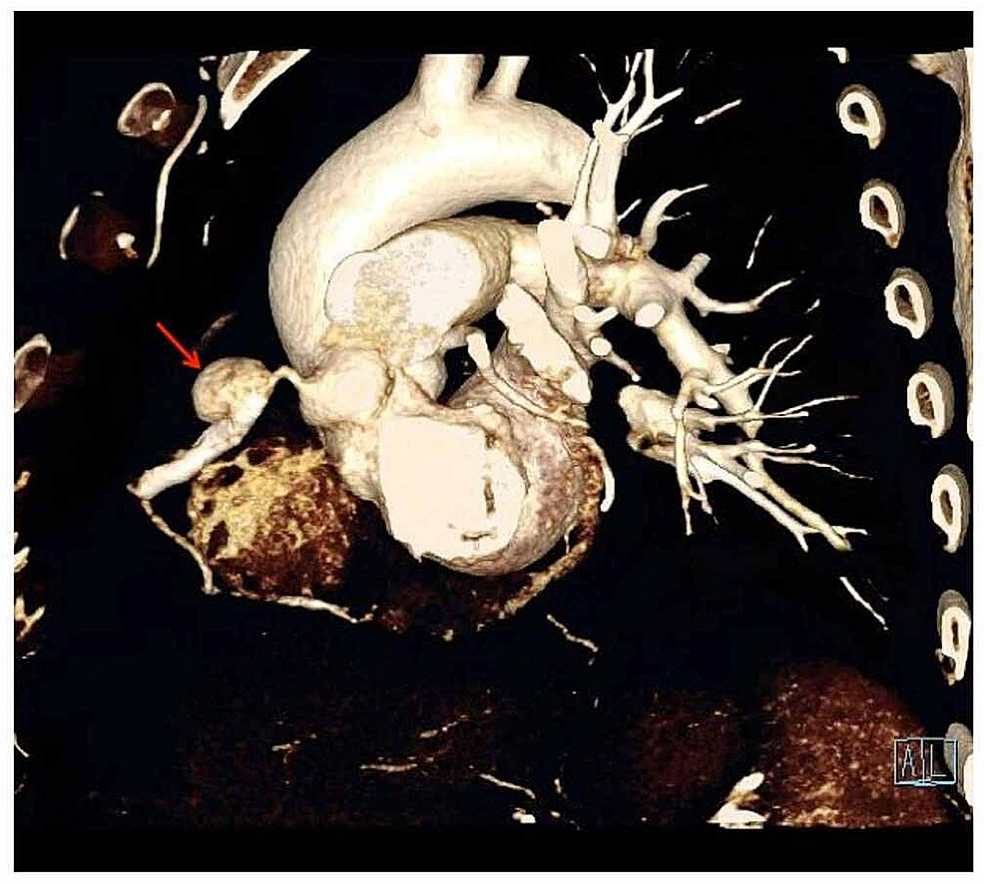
CT coronary angiography: curved volume rendering technique (VRT) image Curved VRT image showing the large pseudoaneurysm at the proximal stent edge (red arrow).
